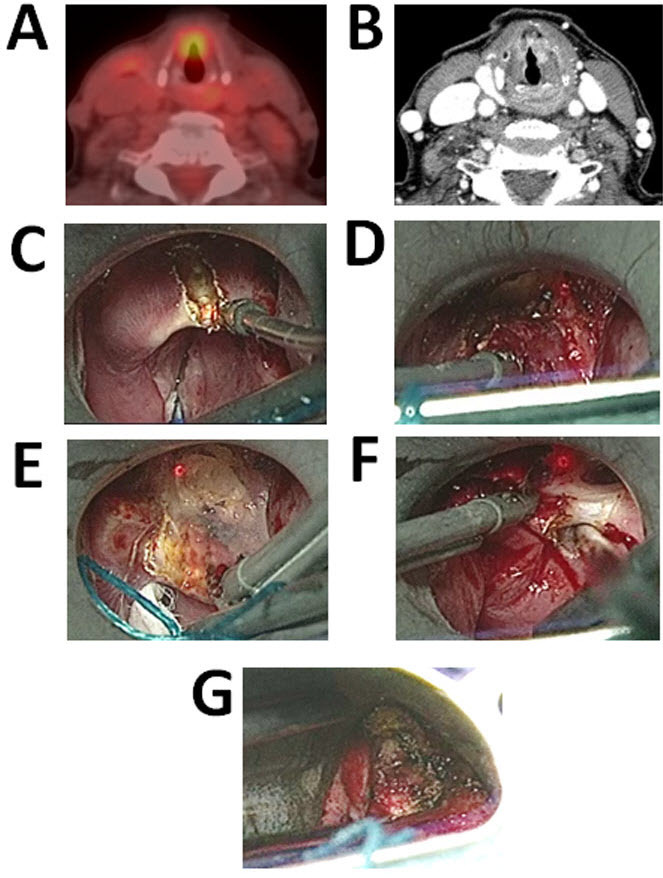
Sixty-six-year-old Female with a History of Laryngeal Carcinoma Treated with EBRT and Chemotherapy Presents with Recurrent Laryngeal Cancer. A: PET scan demonstrating FDG avid lesion of the glottis. B: Pre-operative CT scan demonstrating increased contrast enhancement at the level of the glottis. C–G: Intraoperative photographs. C: The epiglottis is divided in the midline. D: The vascular pedicle is ligated with surgical clips and divided. E: The dissection is carried out anteriorly into the pre-epiglottic space. F: The tumor is released laterally. G: Post partial laryngectomy evaluation of the glottis is performed to insure hemostasis.
